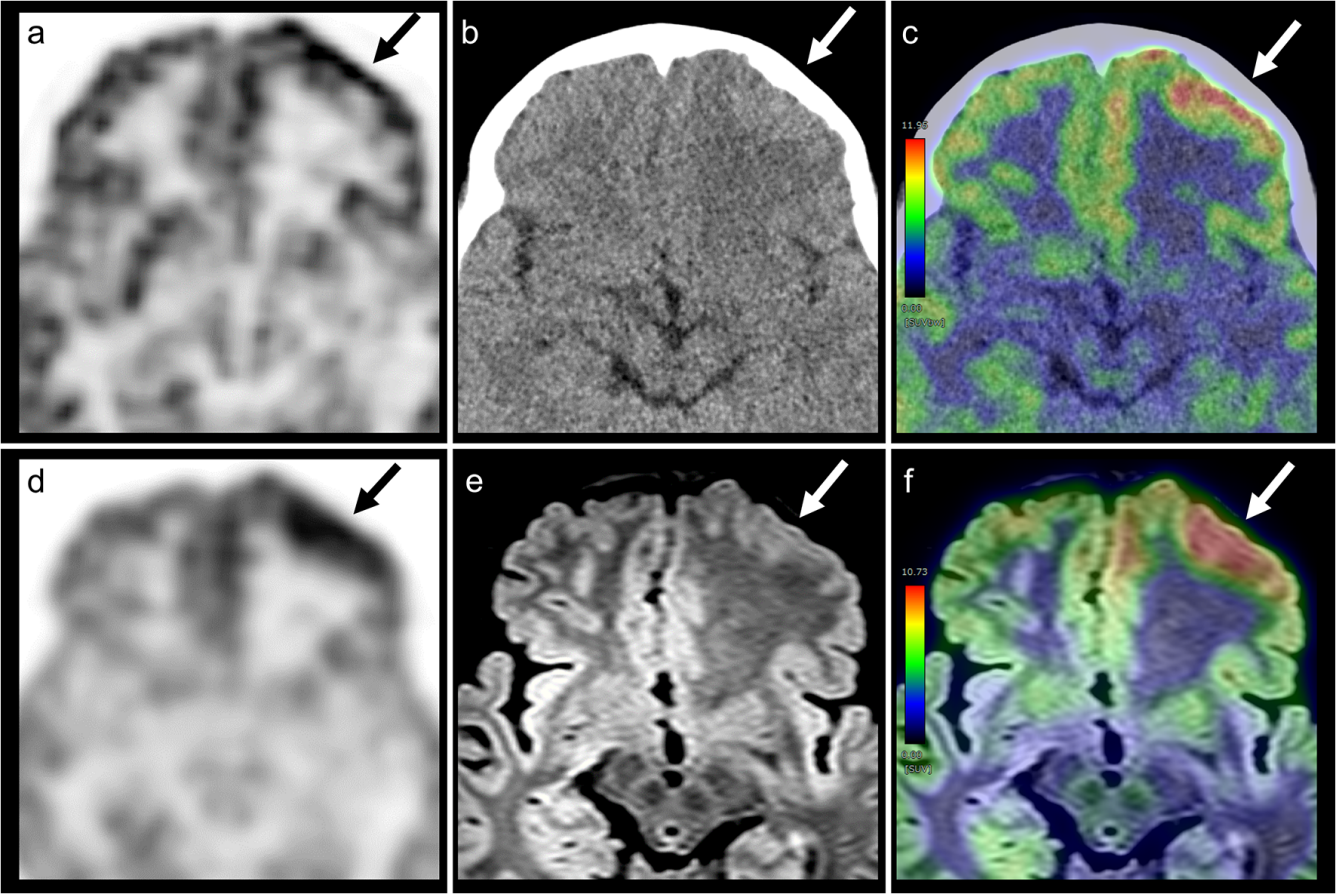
Dedicated PET from PET/CT (a) and PET/MRI (d), CT (b), FLAIR (e), PET/CT (c), and PET/MRI (f) acquired with [18F]-fluorodeoxyglucose (FDG) examinations of the brain in an 8-year-old male with focal cortical dysplasia (FCD), type Ib. A focal area of increased tracer accumulation is shown in the left frontal lobe immediately after ictus, which is consistent with a seizure focus (arrows). Notably, the abnormality of cortical thickness is more clearly depicted on PET/MRI than on PET/CT. After surgery, this patient was completely seizure free (International League Against Epilepsy; ILAE outcome scale 1). *FDG*, fluorodeoxyglucose*; FLAIR*, fluid-attenuated inversion-recovery
